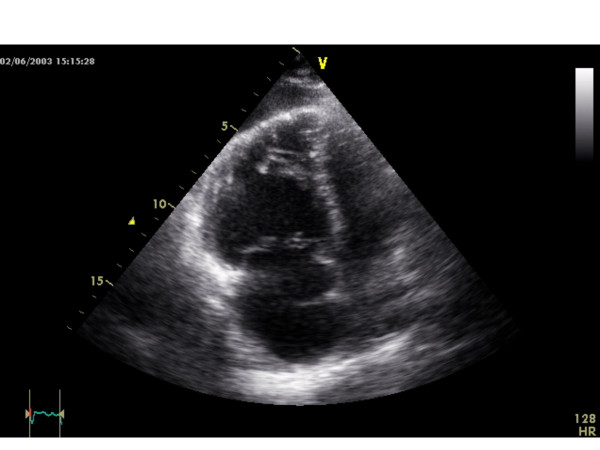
Right ventricular hypertrophy and dilatation at initial investigation with transthoracic echocardiography.
